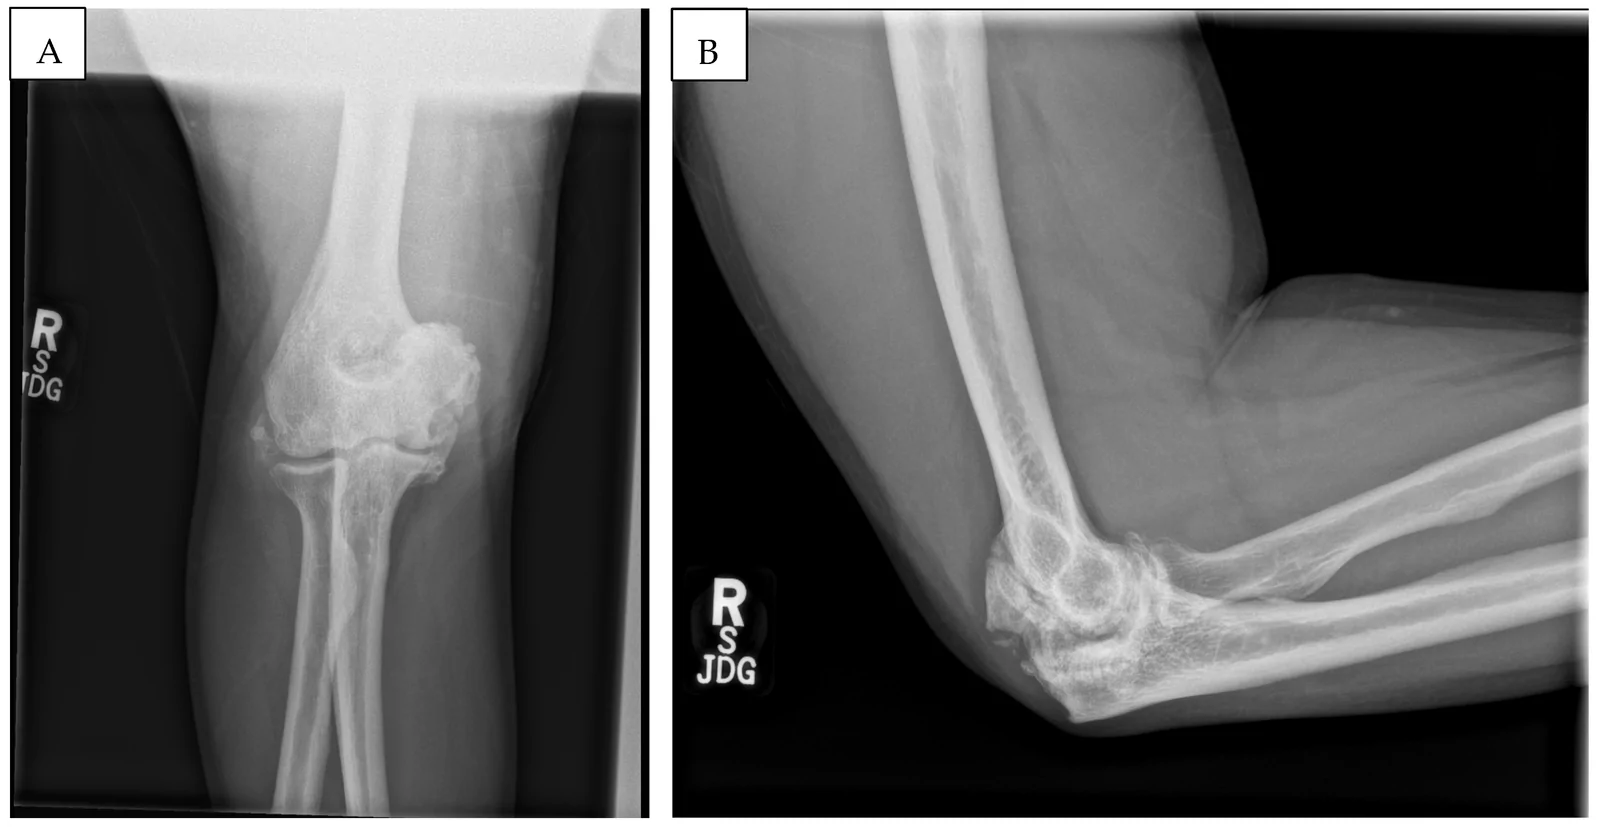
Preoperative (A) anterior to posterior (AP) radiograph. (B) Lateral radiograph. This figure demonstrates severe heterotopic ossification of the right upper extremity at the posteromedial aspect of the humeroulnar articulation upon initial presentation.
